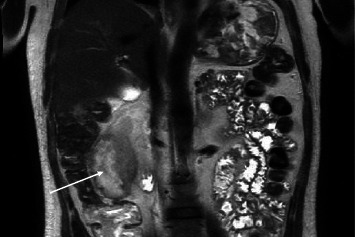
Magnetic resonance imaging of the abdomen showing the perirenal abscess (white arrow).
